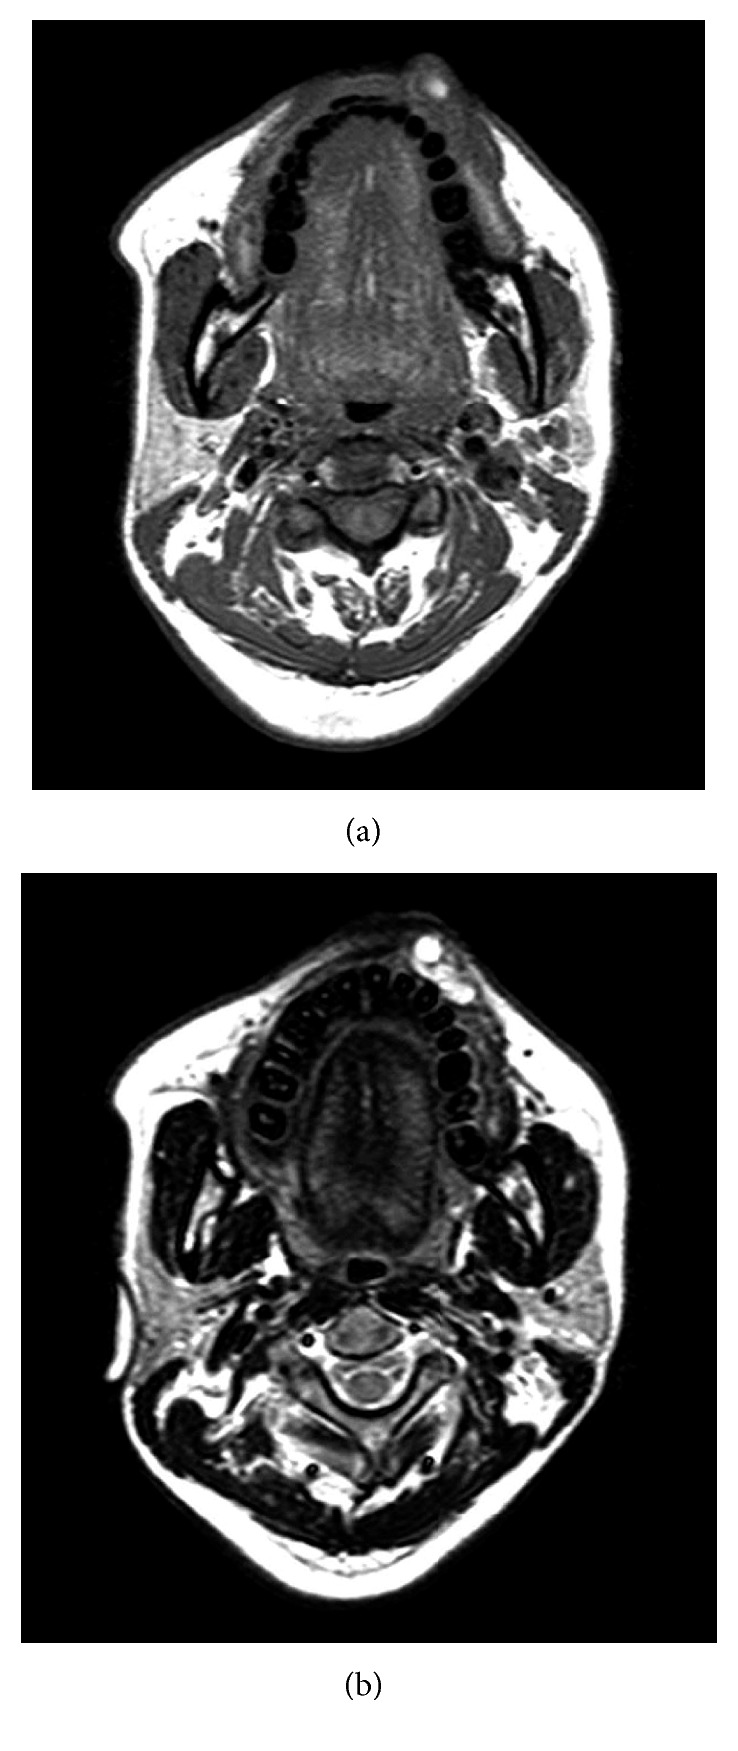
MRI findings. (a) T1-weighted image. (b) T2-weighted image.
